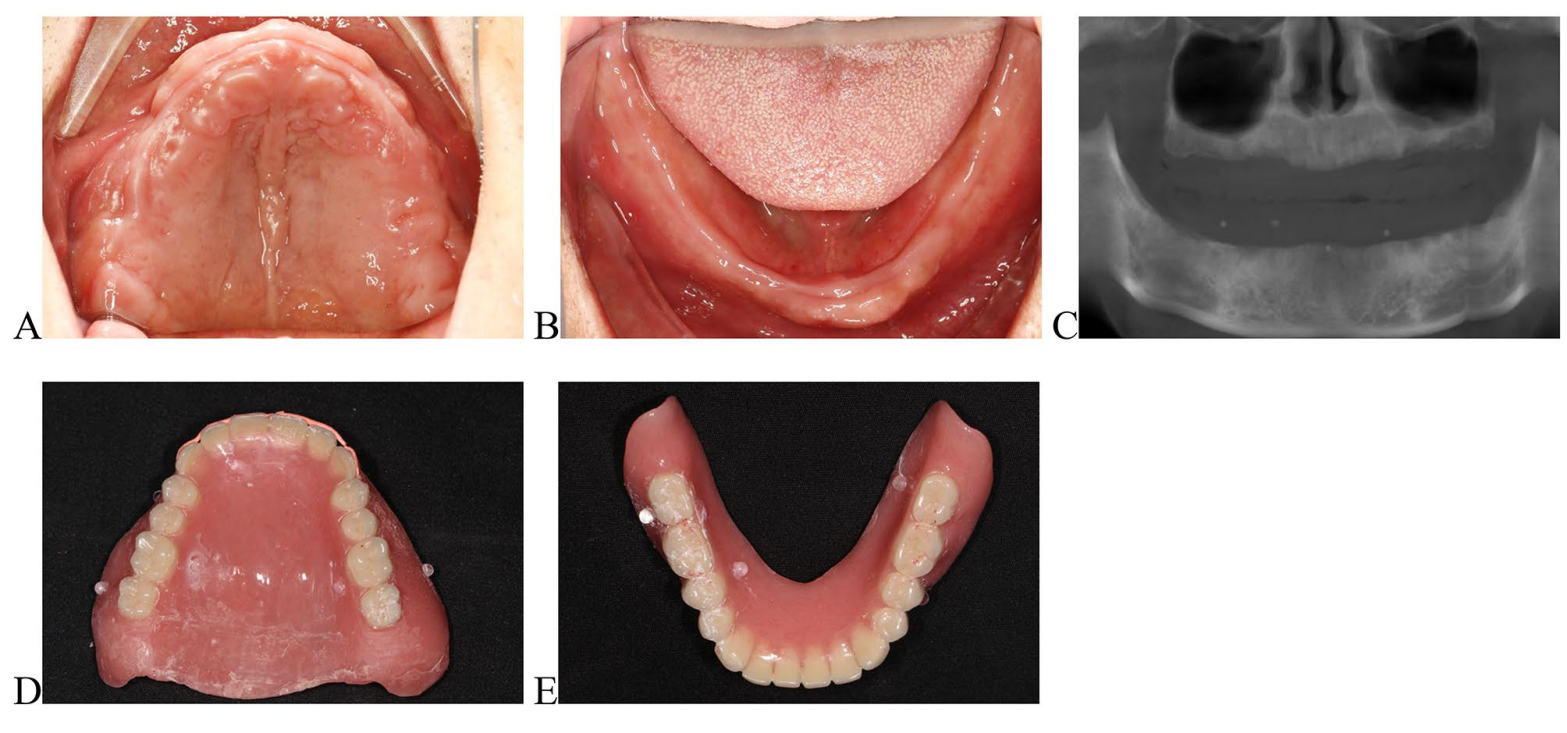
A, Preoperative intraoral photograph of maxillary. B, Preoperative intraoral photograph of mandibular. C, Radiographic examination. D, Maxillary diagnosis denture with smile line. E, Mandibular diagnosis dentureImport the Digital Imaging and Communications (DICOM) files into a computer-aided design (CAD) surgical implant planning program (3Shape 2020), and perform prosthetically driven computer-aided implant planning. Plan four dental implants with four fixation pins in the maxillary and two implants with three fixation pins in the mandible (Template Fixation Pin 1.5 × 32 mm, Inclusive). Import the surgical implant guide information using standard tessellation language (STL) files into the forward engineering software (Materialise 3-matic 20.0) and design the metallic retainer template and implant placement templates (Fig. 2A-D).Fig. 2 Fig2:
